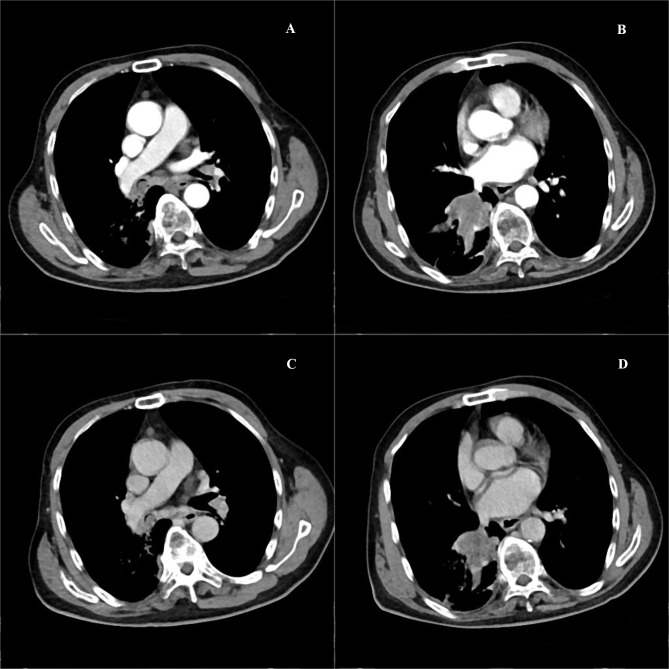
(A–D). Contrast-enhanced chest CT shows an irregular mass at the right lower hilum, extending along the right main bronchus and lower lobe bronchi. The mass displays indistinct boundaries and heterogeneous internal density. It shows progressively irregular enhancement following contrast agent administration.
